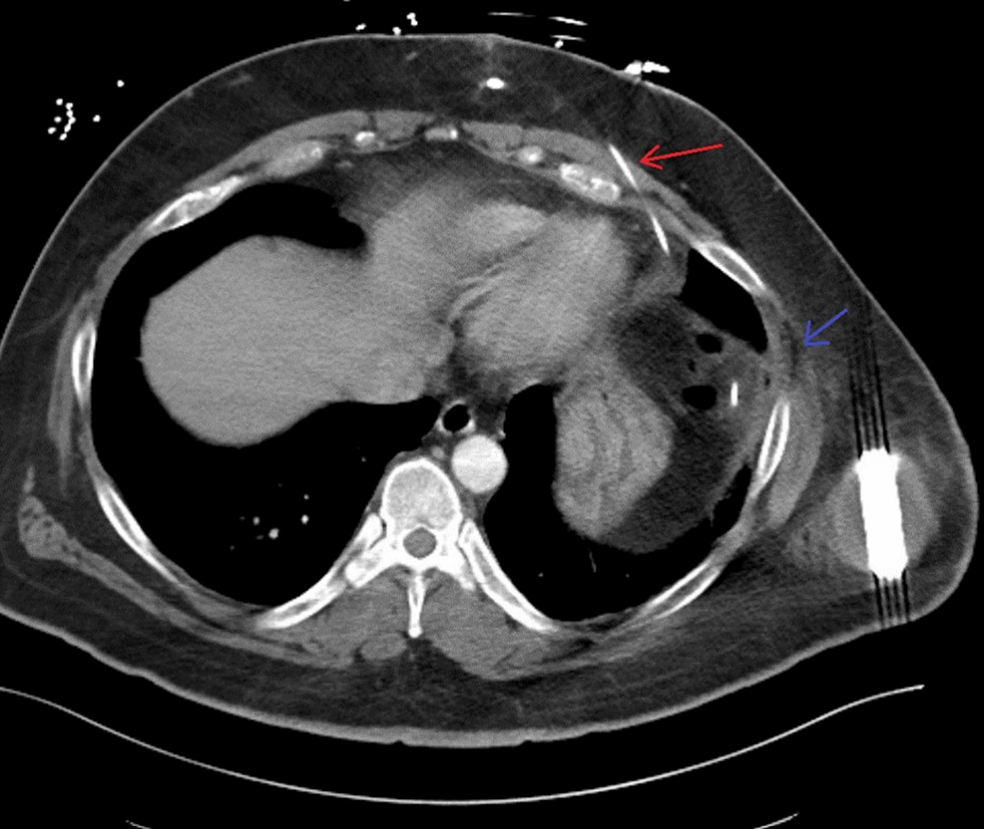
Computed tomography demonstrating the intra-abdominal approach of S-ICD lead traversing the splenic flexure of the colon with the tip (red arrow) located in the anterior subcutaneous tissues of the chest wall and a focus of gas with pleural thickening (blue arrow). S-ICD: subcutaneous implantable cardioverter-defibrillator
